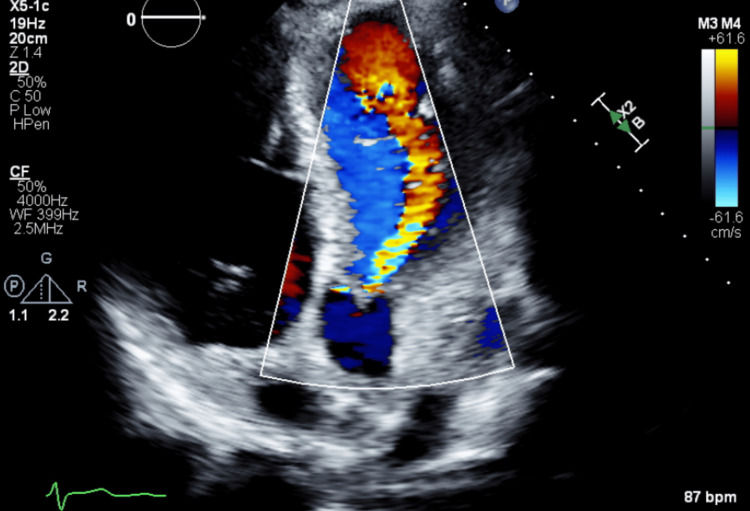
Echocardiography in apical five-chamber view with Doppler demonstrating mild aortic regurgitation
